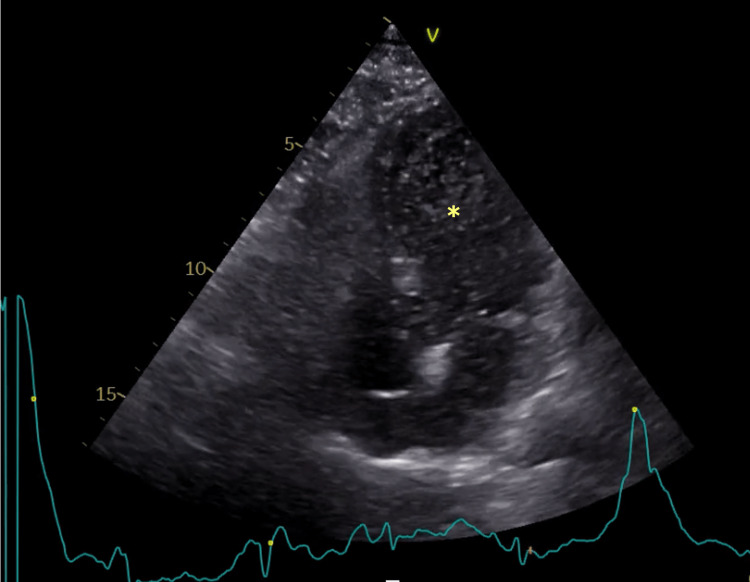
Agitated saline test during a transthoracic echocardiogram. A positive agitated saline/bubble test was observed during the transthoracic echocardiogram, showing bubbles in the left side of the heart (* – left ventricle).
